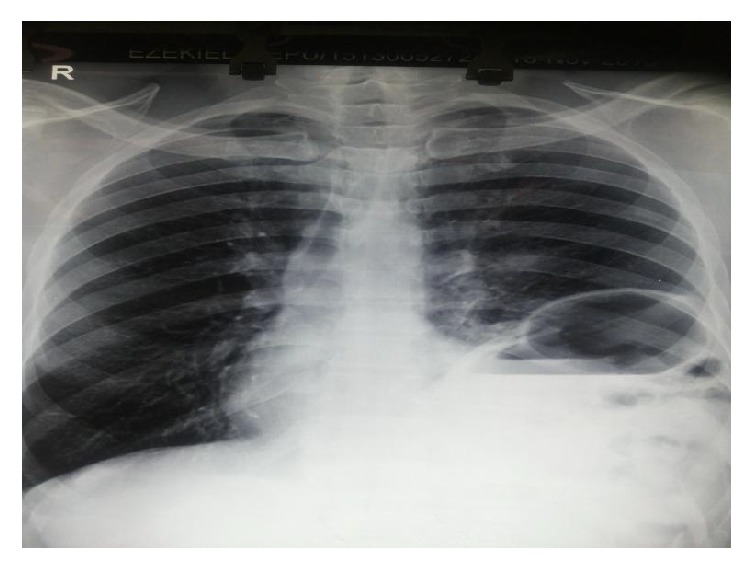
Chest X-ray showing air fluid levels and gastric fundal gas.
